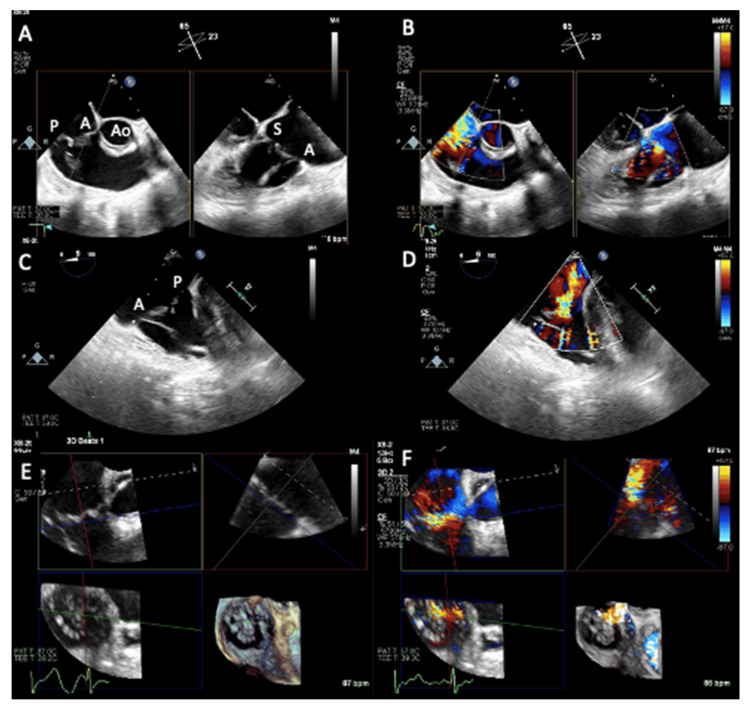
Transesophageal echocardiogram two-dimensional views (A,B): RV inflow-outflow view with biplane imaging; (C,D) deep esophageal 0° view) and multiplanar views (E,F) documenting flail anterior leaflet determining malcoaptation and severe tricuspid regurgitation with color Doppler (B,D,F). Ao = aorta, P = posterior leaflet, A = anterior leaflet, S = septal leaflet.
